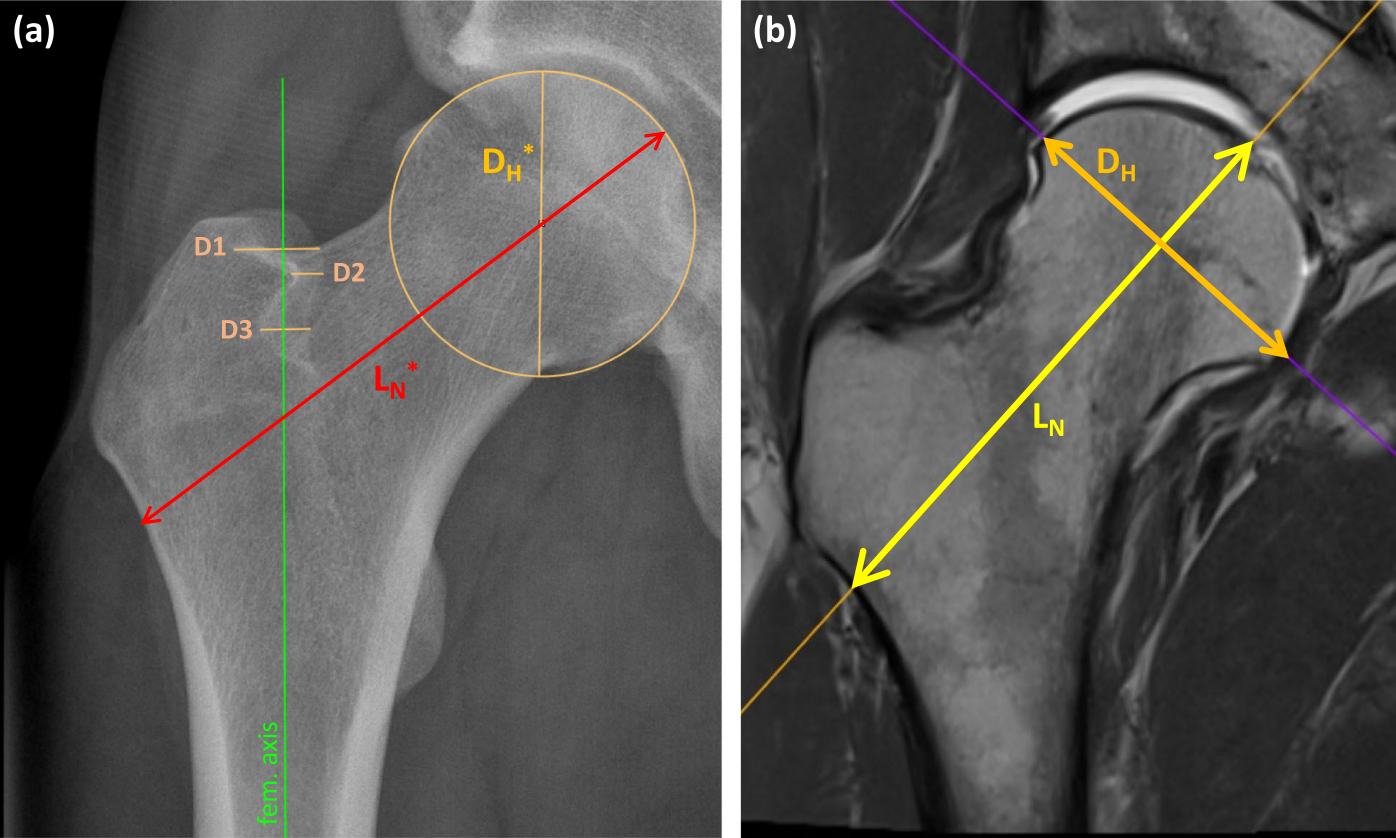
a The apparent femoral neck length, L_N_^*^, was measured on the anteroposterior radiograph as the full bone length spanning the cortical surface of the femoral head to the opposing cortical surface along the femoral neck axis. Apparent femoral head diameter (D_H_^*^) was measured by fitting a circle to the articular surface. b True femoral neck length and femoral head diameter were measured on the magnetic resonance image in the sagittal plane of the femur and compared to the corresponding radiographic measurements
